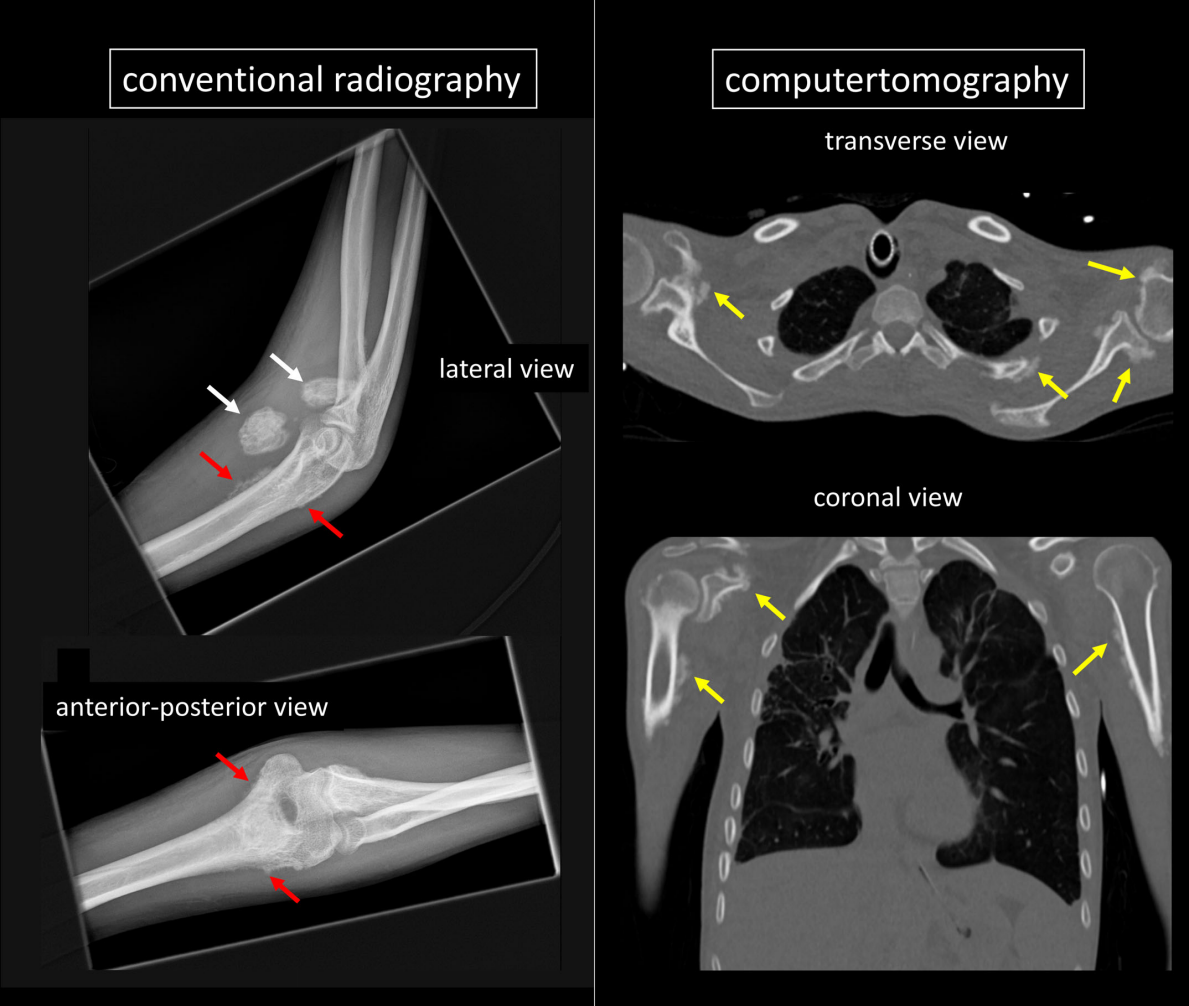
A 25‐year‐old patient was treated with the volatile anesthetic sevoflurane for approximately three months due to agitation unresponsive to intravenous anesthetics. The patient had septic multiorgan failure resulting from a Bartholin abscess and COVID‐19 pneumonia with bacterial superinfection and empyema. Chronic medical conditions included GAD65 antibody–positive autoimmune encephalitis, temporal lobe epilepsy, and Ig deficiency syndrome. The patient developed clinical signs of monoarthritis at the right elbow, raising suspicion for septic or autoimmune arthritis. Arthrosonography revealed severe pericapsulitis without distinct synovitis and joint effusion. Arthrocentesis yielded no fluid. X‐ray imaging demonstrated signs of calcifying periostitis (red arrows), amorphous calcifications at the medial aspect of the joint capsule (white arrows), and altered trabeculation in the juxtaarticular distal humerus. A subsequent chest computed tomography identified periostitis with new bone formation of ribs two to eight bilaterally, both scapulae, and both proximal humeri (yellow arrows). Fluoride levels were elevated in serum (1.62 mg/L; normal <0.1 mg/L) and urine (47.0 mg/L; normal <1.0 mg/L). This led to a diagnosis of sevoflurane‐induced skeletal fluorosis. Three weeks after discontinuing sevoflurane, clinical and ultrasound signs regressed, and fluoride levels in serum (0.24 mg/L) and urine (1.7 mg/L) dropped. Unfortunately, the patient died soon thereafter due to refractory septic shock. Approximately 5% of inhaled sevoflurane are metabolized to hexafluoroisopropanol with release of inorganic fluoride and carbon dioxide.^1^ Although skeletal fluorosis from environmental exposure^2^ and long‐term voriconazole exposure^3^ is recognized, to our knowledge, this is the first reported case associated with volatile anesthetics. Informed consent could not be obtained because the patient was deceased. All efforts were made to ensure that no personally identifiable information was disclosed.
